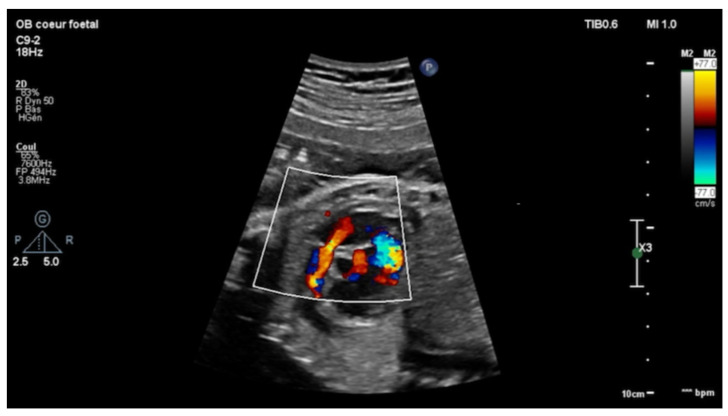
Short-axis view: color Doppler: retrograde flow in the pulmonary artery with pulmonary insufficiency.
